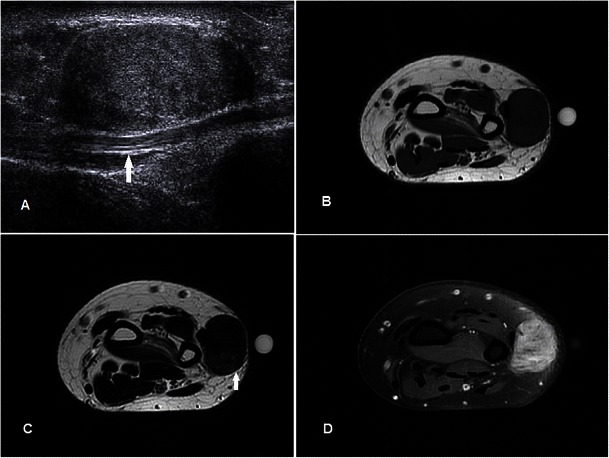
Fibrosarcoma of the tendon sheath in a 47-year-old female presenting with focal swelling along the ulna aspect of the right wrist, gradually increasing in size over a few months. (a) Ultrasound shows a well-defined hypoechoeic lesion along the ulnar side of the wrist abutting the extensor carpi ulnaris (arrow). (b) T1w sequence shows a hypointense well-circumscribed subcutaneous lesion abutting the extensor carpi ulnaris (arrow). (c) The lesion is predominantly of low signal on T2w sequence, with small areas of high signal (arrow) indicating a more cellular/necrotic component. (d) Heterogeneous enhancement is seen, in contrast to a benign FTS (Fig. 3), which shows only minimal enhancement
